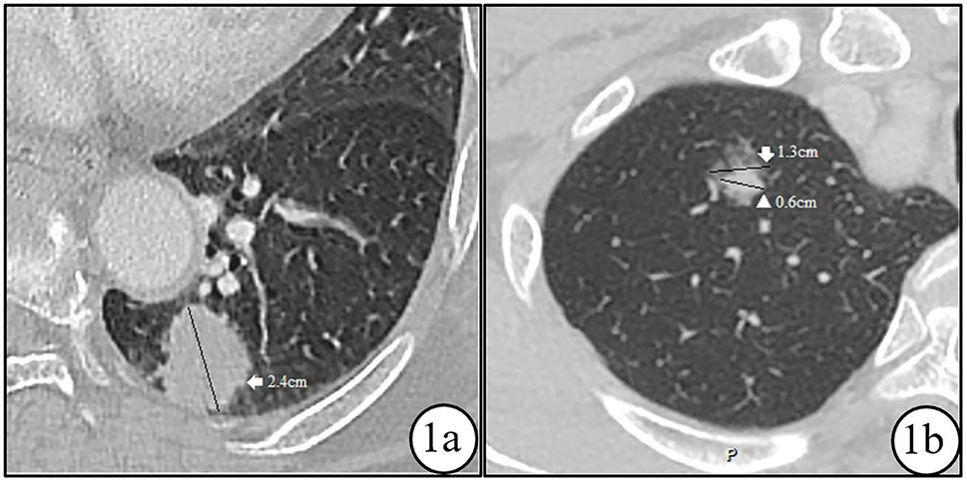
a shows a female patient aged 58 with a solid nodule in the lower lobe of the left lung, as seen on CT scan with a maximum diameter of 2.4 cm (indicated by the white arrow). The nodule has a CTR of 1. Similarly, in b, a male patient aged 61 is shown to have a partially solid nodule in the upper lobe of the right lung, with a solid component size of 0.6 cm (indicated by the white arrowhead) and a maximum diameter of 1.3 cm (indicated by the white arrow). The CTR for this lesion is 0.6/1.3 = 0.46
